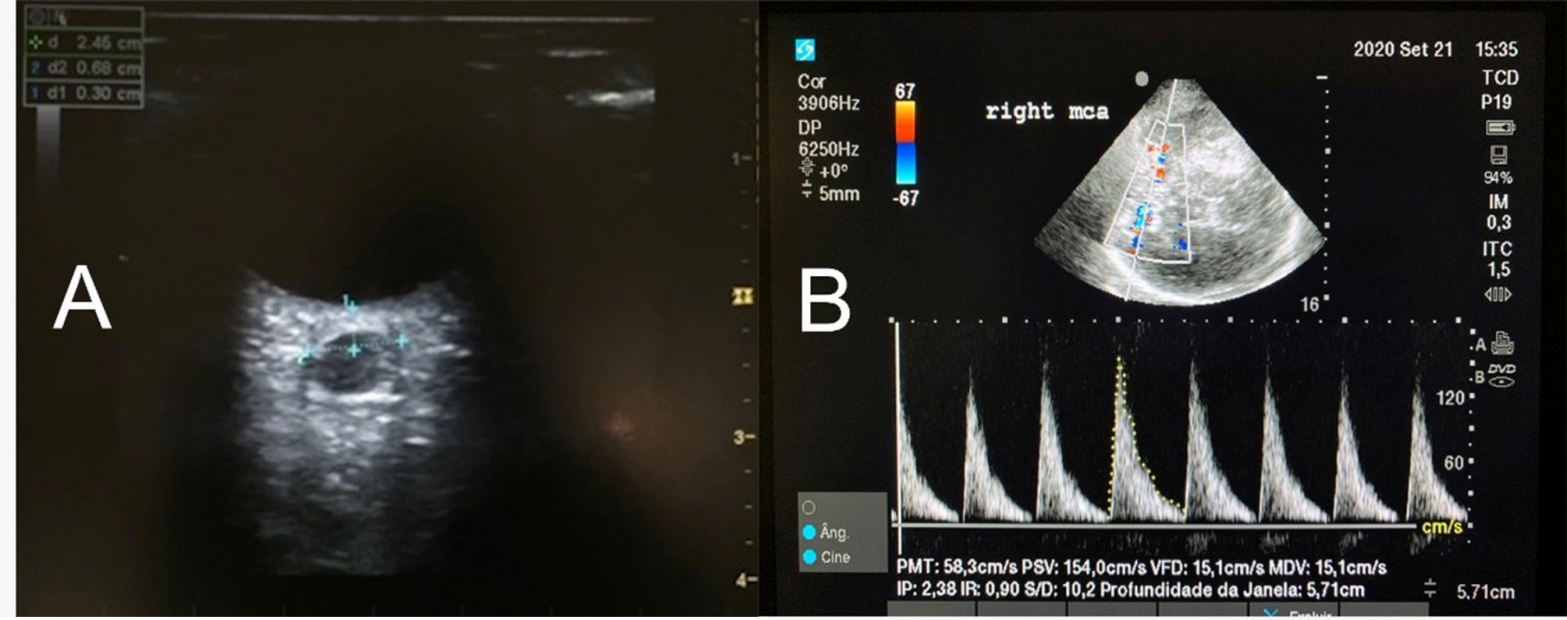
(A) The axial image of the optic nerve sheath diameter (ONSD) in a patient with increased ICP. The ONSD is measured with a linear transducer placed over the closed eye and the measurement of the ONSD is performed perpendicularly to an electronic caliper positioned 3 mm behind the retina. The same measurement is performed at least 3 times (or more, if the variation in between is beyond 10%). Each eye will have a mean value. (B) MCA waveform with color Doppler. PSV, Peak systolic velocity, in cm/s. VDV, End diastolic velocity, in cm/s. Note the Pulsatility Index (IP) suggesting high ICP.
